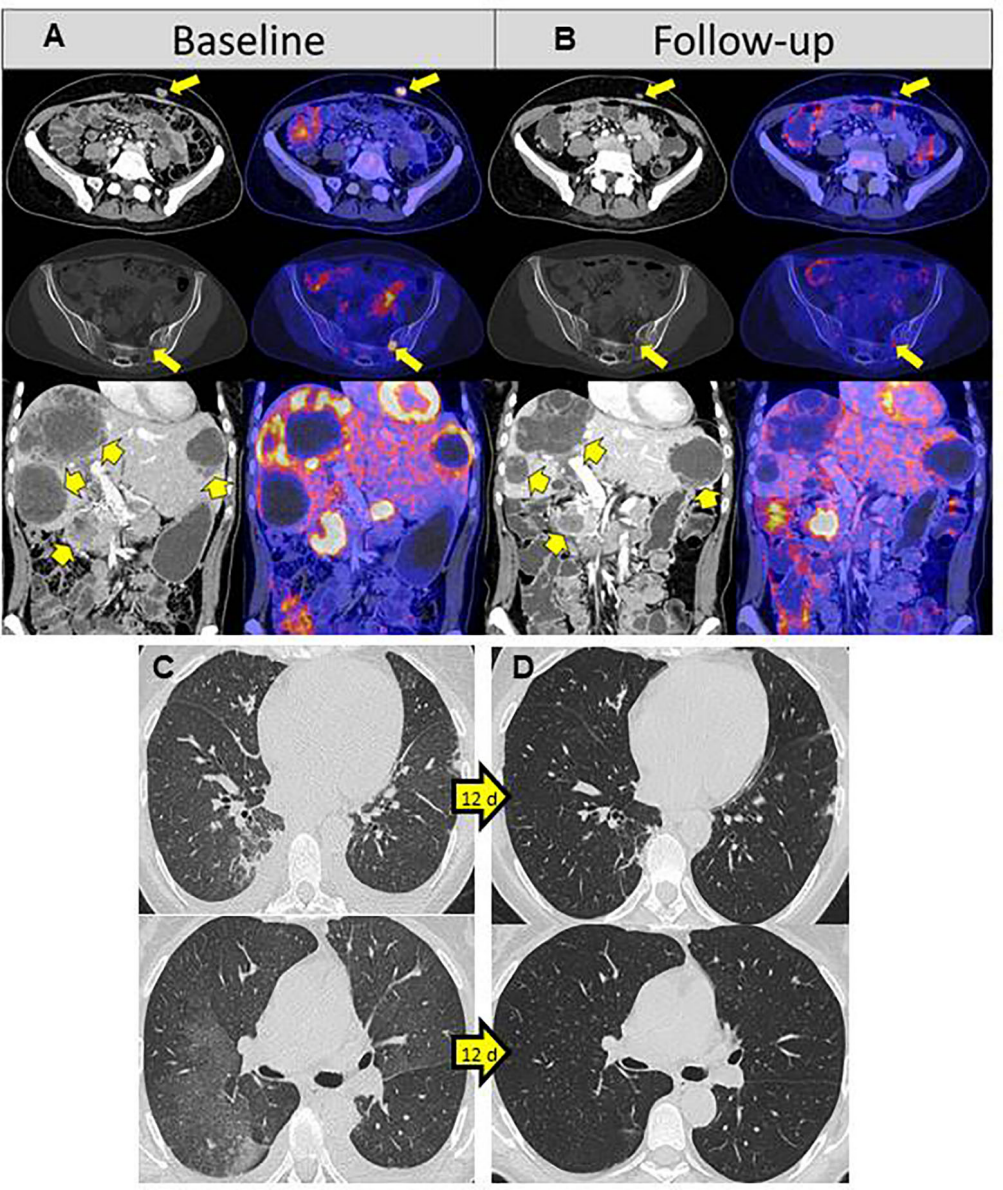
^18^F-FDG PET/CT baseline and 8 weeks of treatment with ribociclib and binimetinib (A, B). (A)
